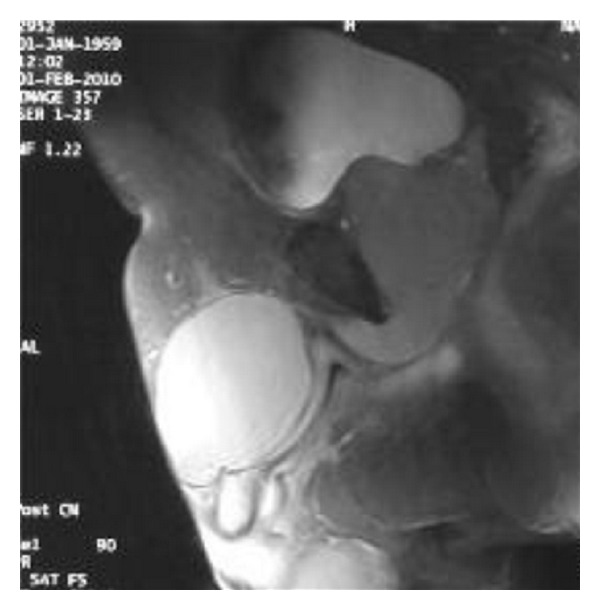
MRI showing the accessory bladder in front of the normal bladder.
